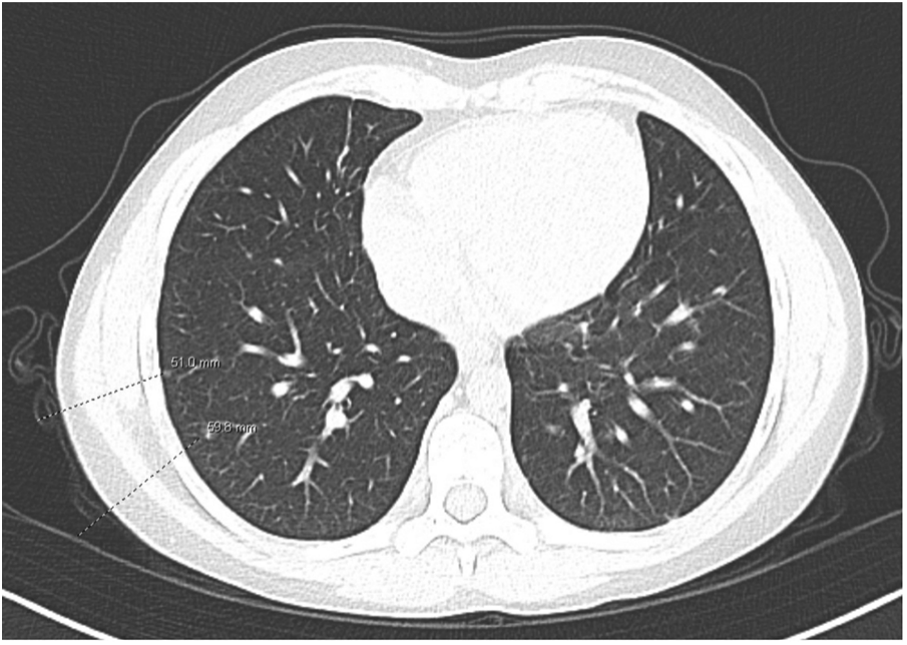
CT following the use of steroids. Mixed response in multiple pulmonary nodules, with some nodules having resolved and other nodules new.
